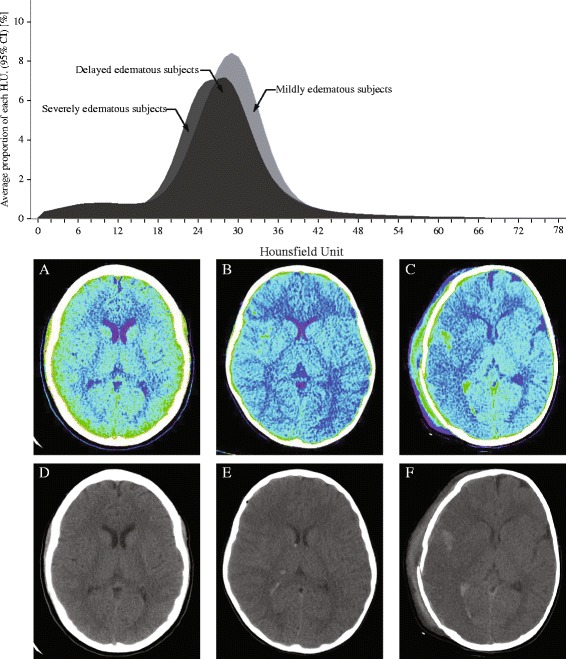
Density histograms from initial CT images of TBI subjects. Notice that two histograms (severe TBI subjects and subjects with delayed cerebral edema) show different distributions from mild TBI subjects. Color-mapped CT images are from (A) a mild TBI subject, (B) a subject who developed delayed cerebral edema, and (C) a severe TBI patient. (D), (E), (F) are their corresponding raw CT images. CT, computed tomography; TBI, traumatic brain injury.
